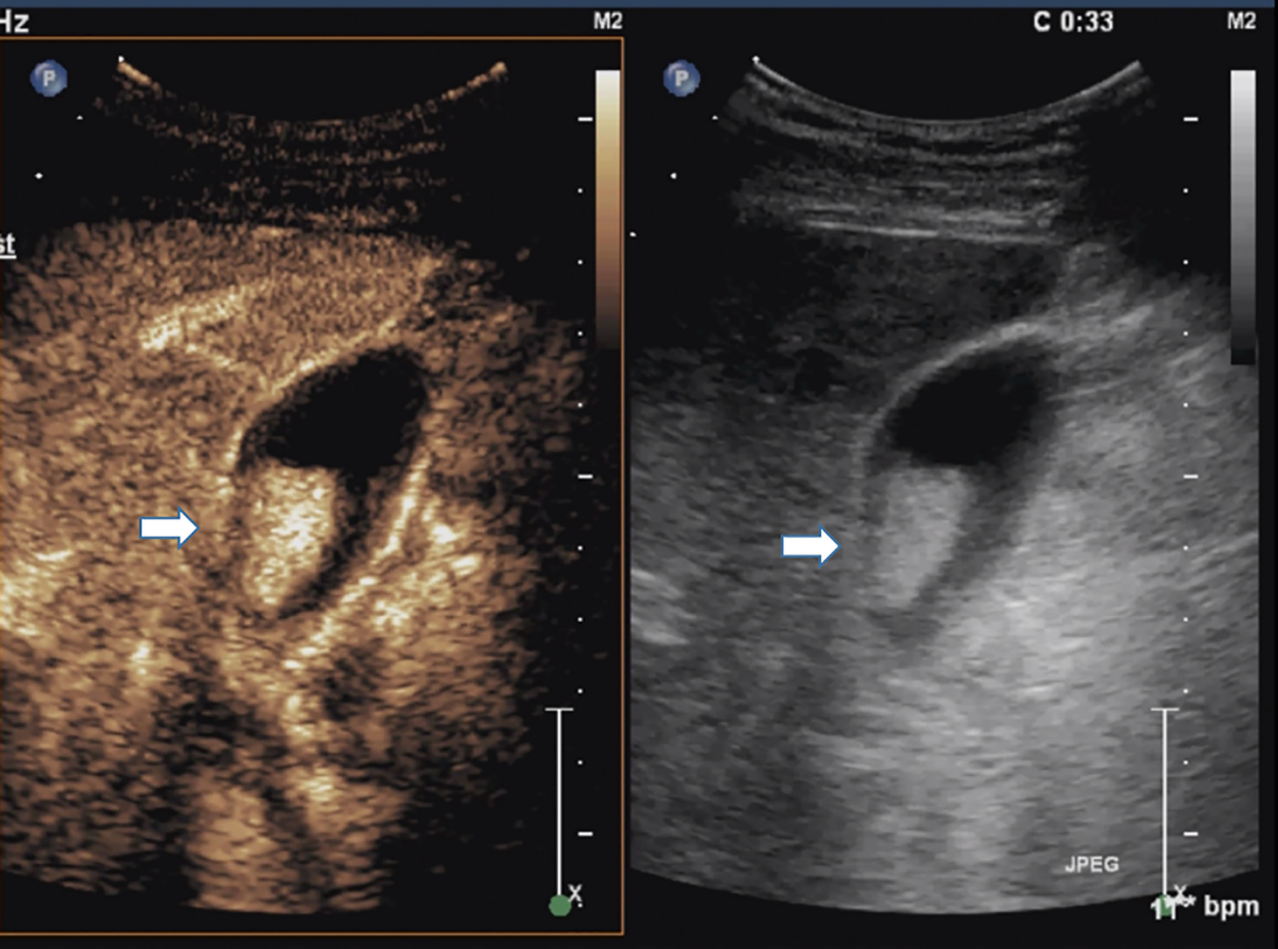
Cholesterol polyp measuring 22 mm in diameter in a 51-year-old man. Right, Conventional sonogram 2D mode (arrow). Left, Microvascular image of CEUS showing intralesional vessels with a strong and heterogeneous pattern. (arrow). So it was mistaken as gallbladder cancinoma.
